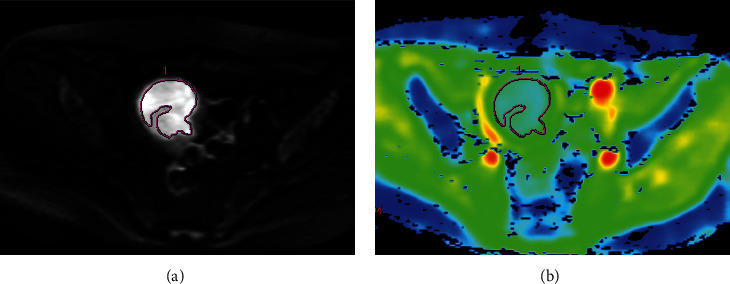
DWI image of a 57-year-old patient with EC of stage IB. (a) ROI was outlined at the largest tumor cross section on DWI (*b* = 1000 s/mm^2^). (b) ADC pseudocolor image showed that the ADC was 0.966 × 10^−3^ mm^2^/s.
